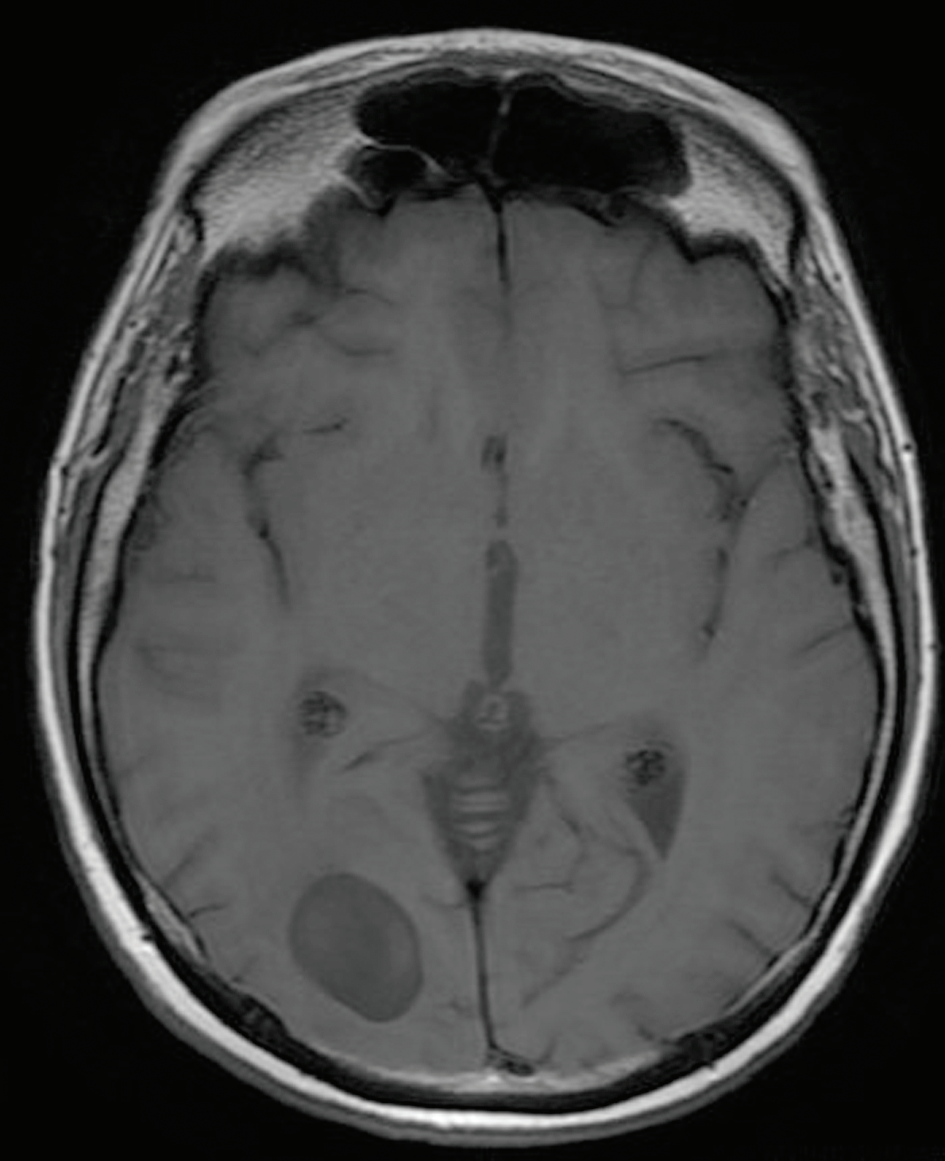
Pre-operative brain MRI T1 sequence.
